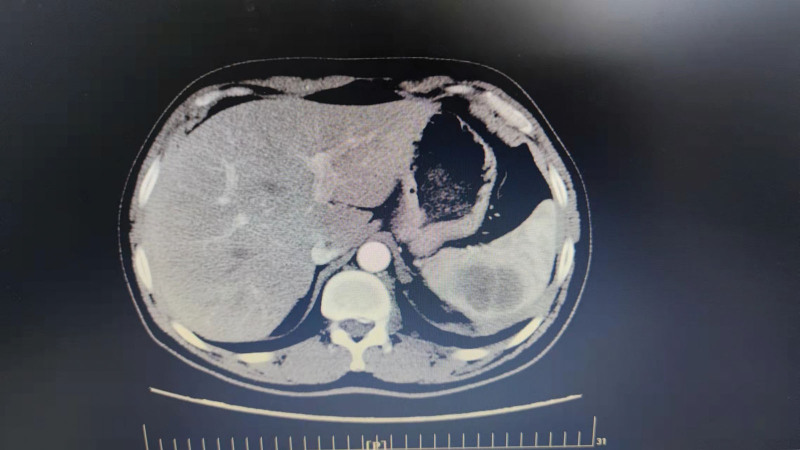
Computed tomography (CT) scan of a patient with splenic metastasis from rectal carcinoma.
